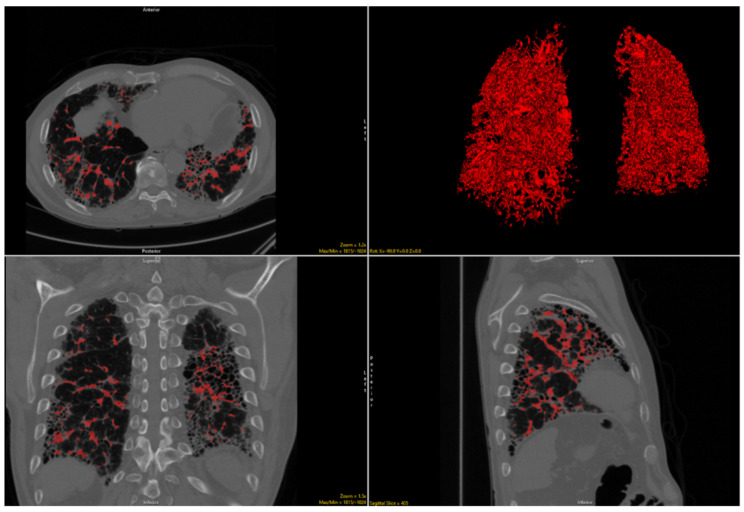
Delineation of volume-related structures on noncontract chest CT images and 3D reconstruction in a patient with idiopathic pulmonary fibrosis.
